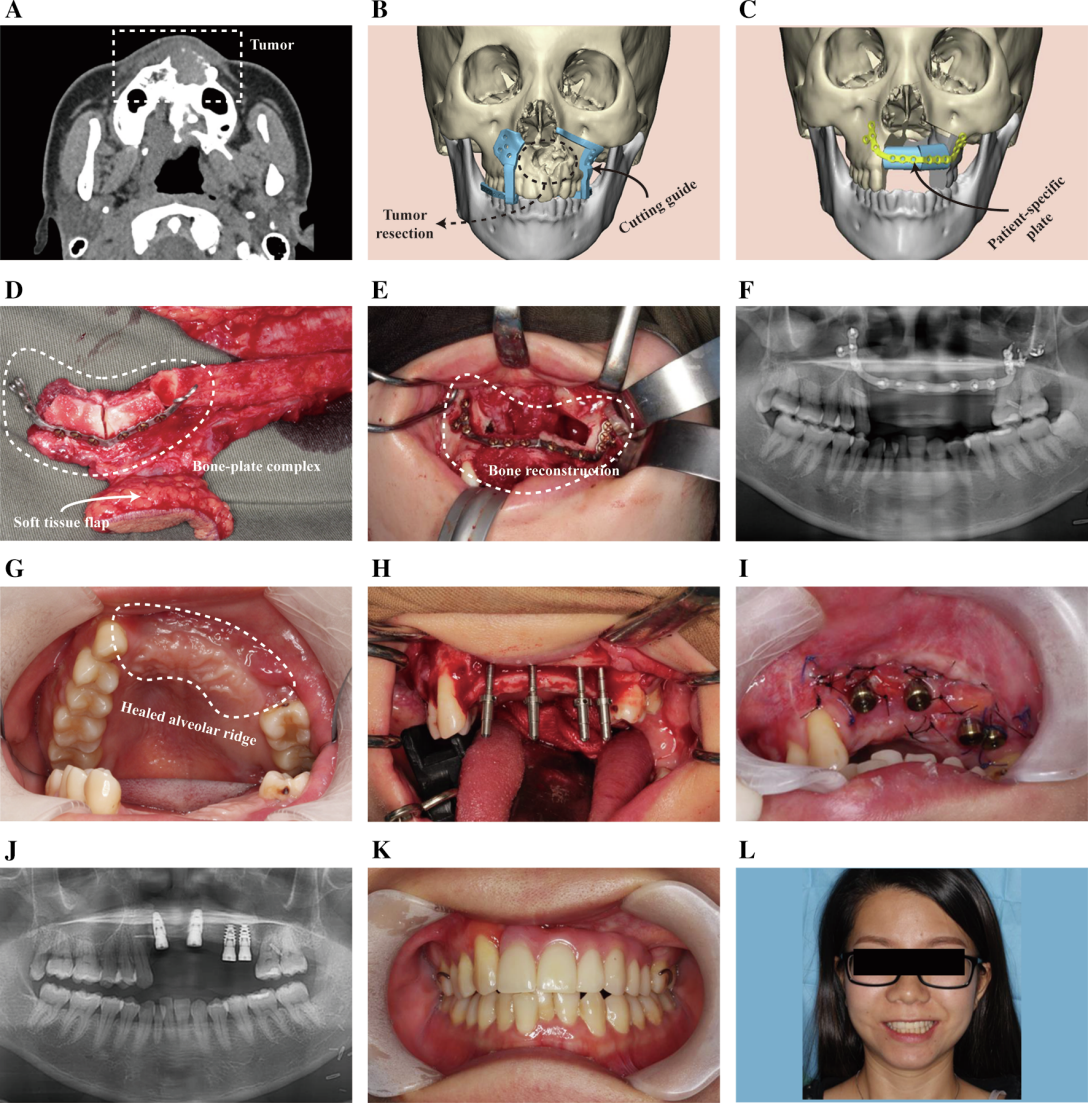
A 33-year-old woman with desmoplastic ameloblastoma in the anterior maxilla underwent surgery. a The preoperative computed tomography (CT) image indicates the destructive mass in the anterior maxilla. b A three-dimensional (3D) virtual model is used to delineate bone resection margins and design cutting guides. c The patient-specific surgical plate is designed to fix bone grafts. d The vascularized fibular flap is harvested, segmented, folded, and fixed in alignment with the patient-specific surgical plate. e The bone-plate complex is transferred to repair the defect site. f Postoperative OPG showing satisfactory bone healing. g Postoperative intraoral image showing healed alveolar ridge. h Dental implants placed in the transplanted fibula in the second stage. i Intraoral image showing the accurate position of implants as planned. j OPG showing satisfactory implant position and angulation. k Immediate loading of dental implants supports the removable partial denture in the anterior maxilla. Excellent occlusal relationship is achieved. l Satisfactory postoperative aesthetics
